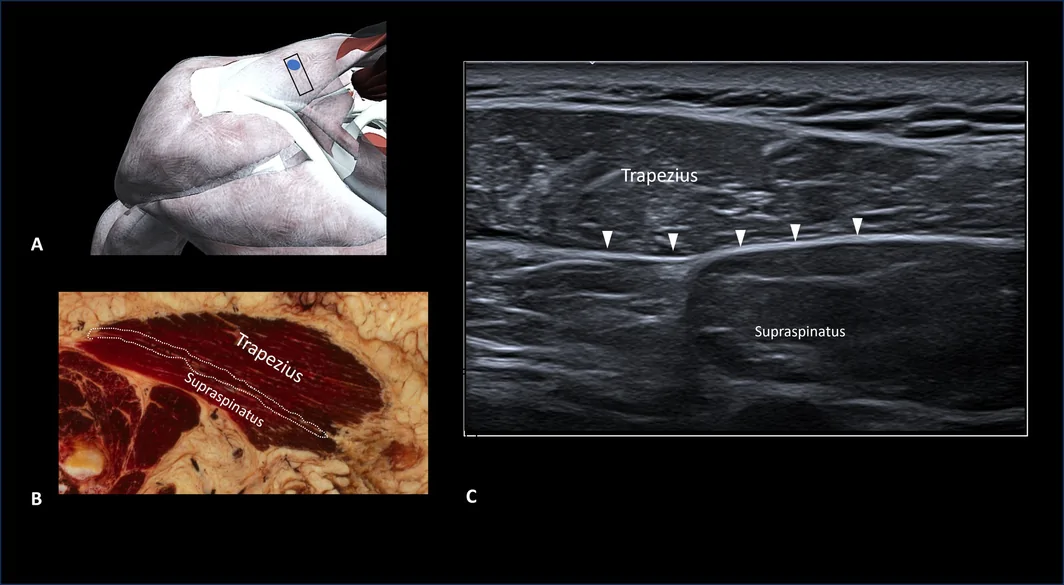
Supraspinatus fascia. A, Probe positioning. B, Coronal anatomical section derived from the Visible Human Project®. *Courtesy of the U.S. National Library of Medicine (NLM), National Institutes of Health* (dotted line: supraspinatus fascia). C, Ultrasound image revealing the supraspinatus fascia (arrowheads).
